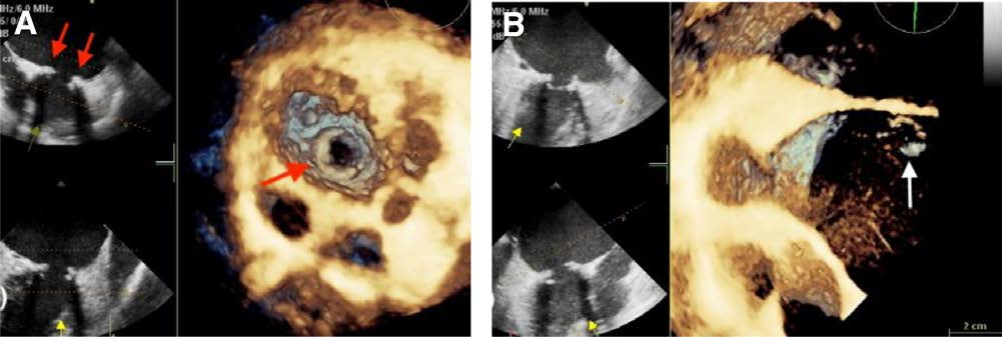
(A) Perioperative 3D-TEE images, looking from the left ventricle toward the mitral valve, depicted elevated tissue around the mitral position without forming a complete ring. (B) 3D-TEE revealed the complete prosthesis ring echo in the left atrium. 3D-TEE = three-dimensional real-time transesophageal echocardiography.
